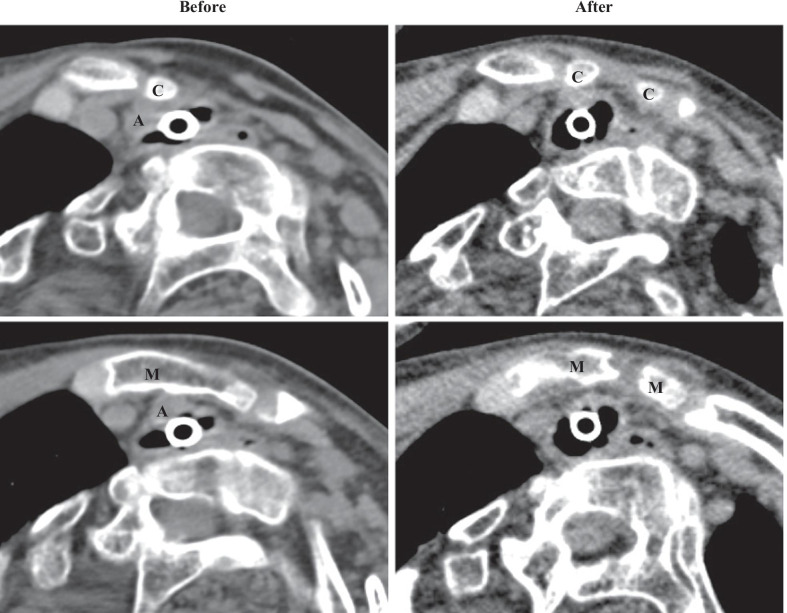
Computed tomography imaging before and after transection of the brachiocephalic artery and thoracoplasty in Patient 2. * A* brachiocephalic artery;* C* sternal end of the clavicle;* M* manubrium sterni
